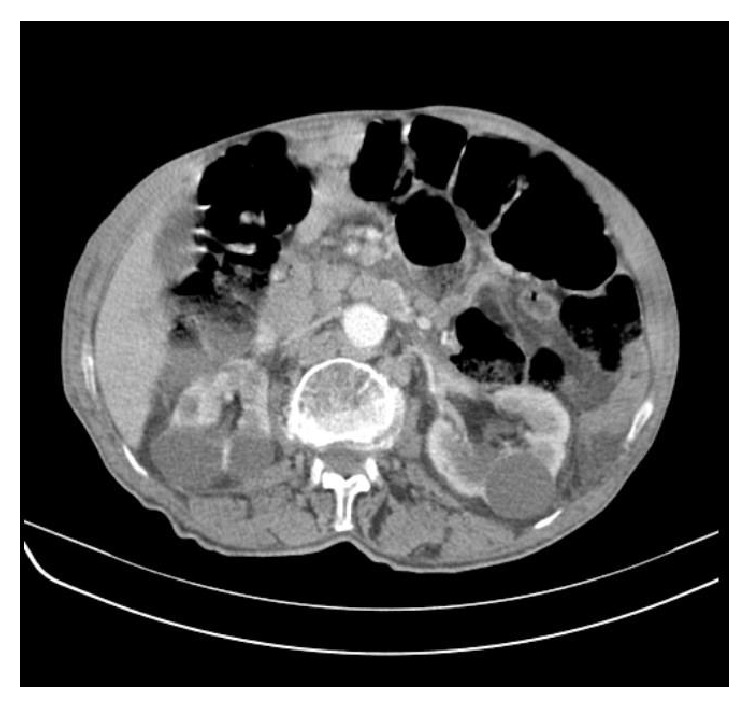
Abdominal CT demonstrating multiple enlarged lymph nodes in the retroperitoneum. The patient also presented bilateral renal cysts.
